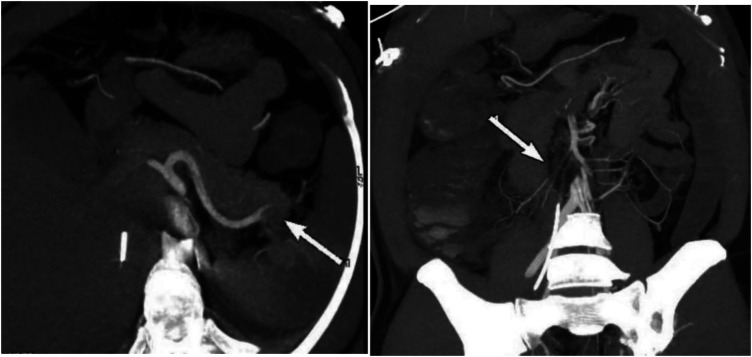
Preoperative CTA showed an acute completely embolic occlusion of arteria mesenterica superior and splenic artery (arrow).
